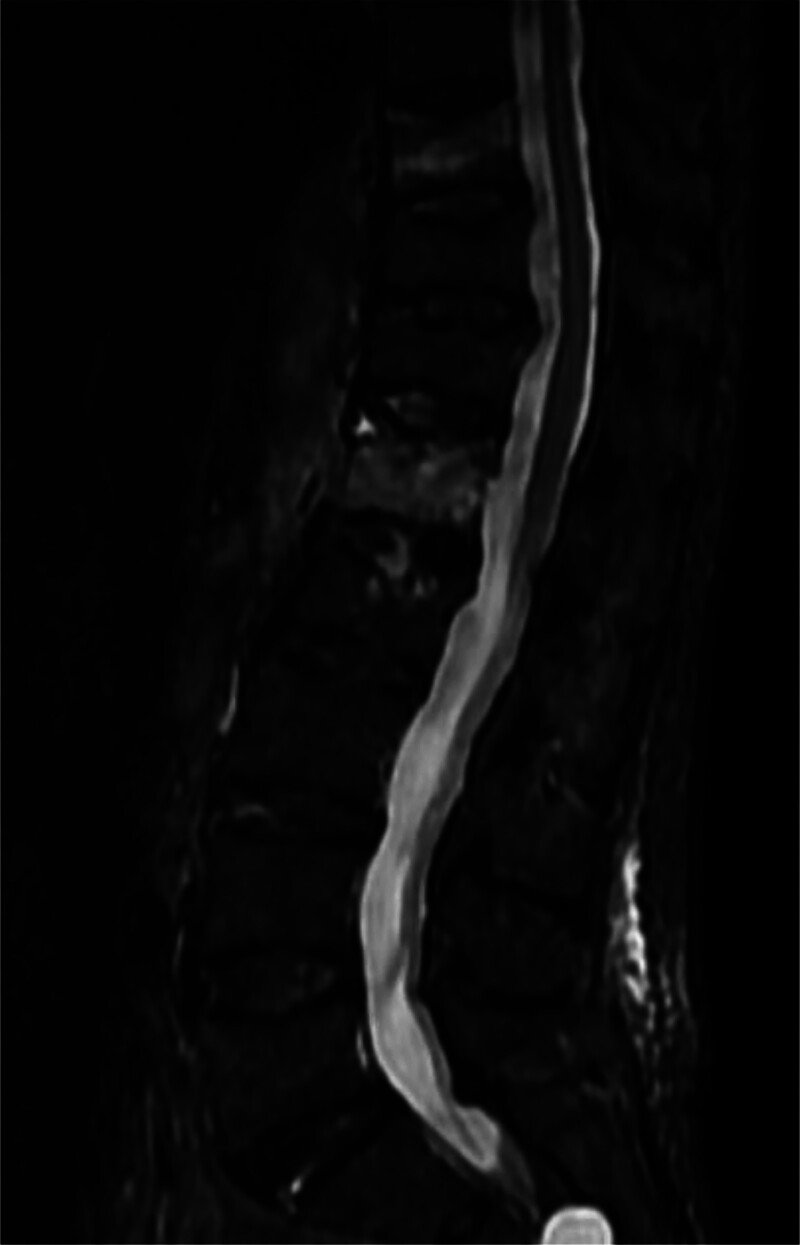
Preoperative MRI demonstrating T10 and L1 vertebral compression fractures.
